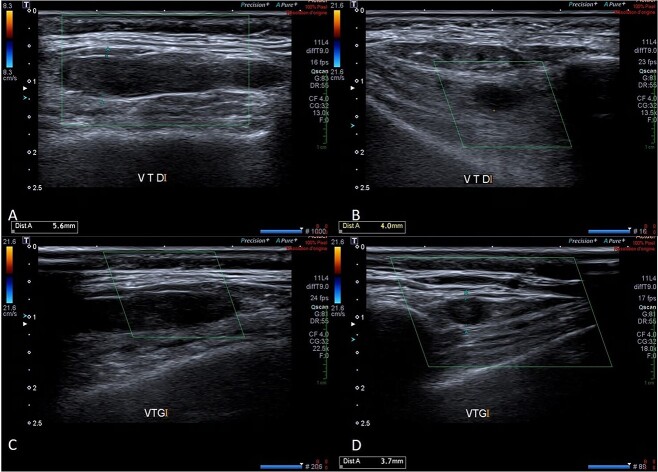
Doppler ultrasound images taken during temples examination. A: Longitudinal section of thrombus approximately 30 mm long in the right superficial temporal vein. B: Transversal section of the thrombus in the right superficial temporal vein, 4 mm thick. C: Longitudinal section of a thrombus over 20 mm long in the left superficial temporal vein. D: Cross-section of the thrombus in the left superficial temporal vein, thickness measured at 3.7 mm.
